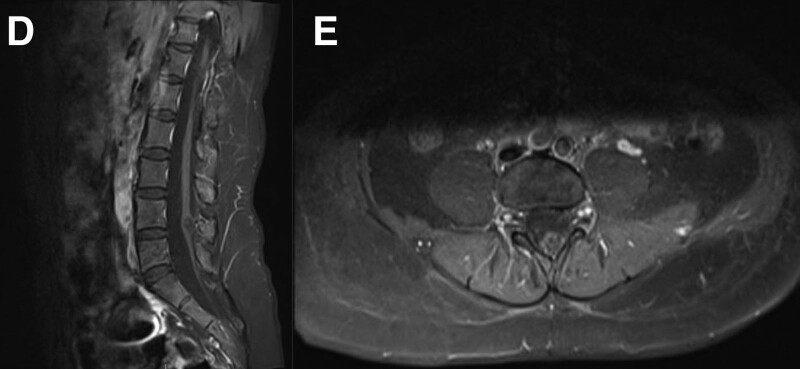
(D and E) Preoperative lumbar spine enhanced MRI scans in the sagittal plane and cross-section, respectively. MRI = magnetic resonance imaging.
